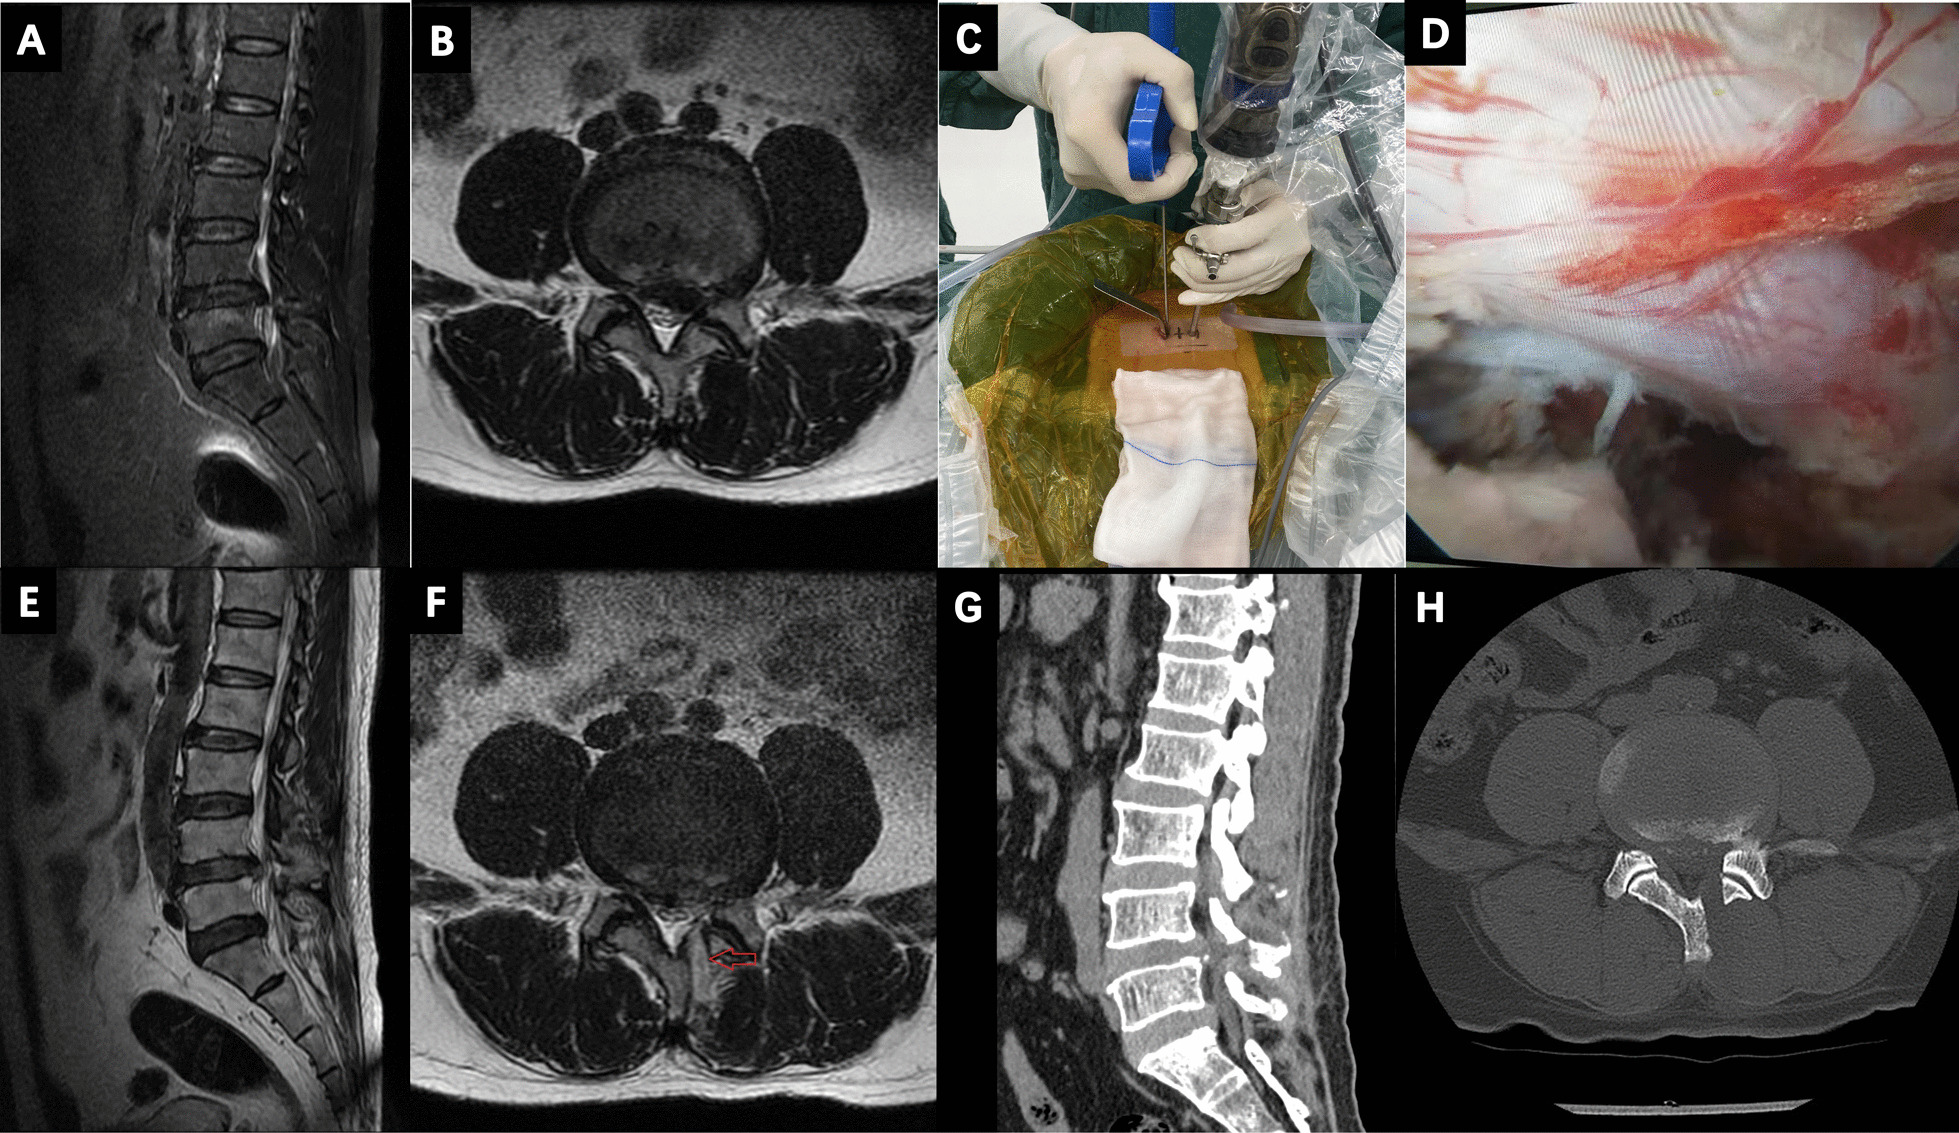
A, B Preoperative sagittal and axial magnetic resonance images in a 34-year-old male patient complaining of left radicular leg pain, showing L4–L5 disc herniation on the left side. C Image of the patient who underwent unilateral biportal endoscopic discectomy. D Endoscopic image showing the relaxation of L5 nerve root after decompression. E, F Postoperative sagittal and axial magnetic resonance images made one day after surgery. High signal intensity (red arrow) indicating blood extravasates. G, H Postoperative computed tomography made two days after surgery showing defect of lamina after partial laminotomy
